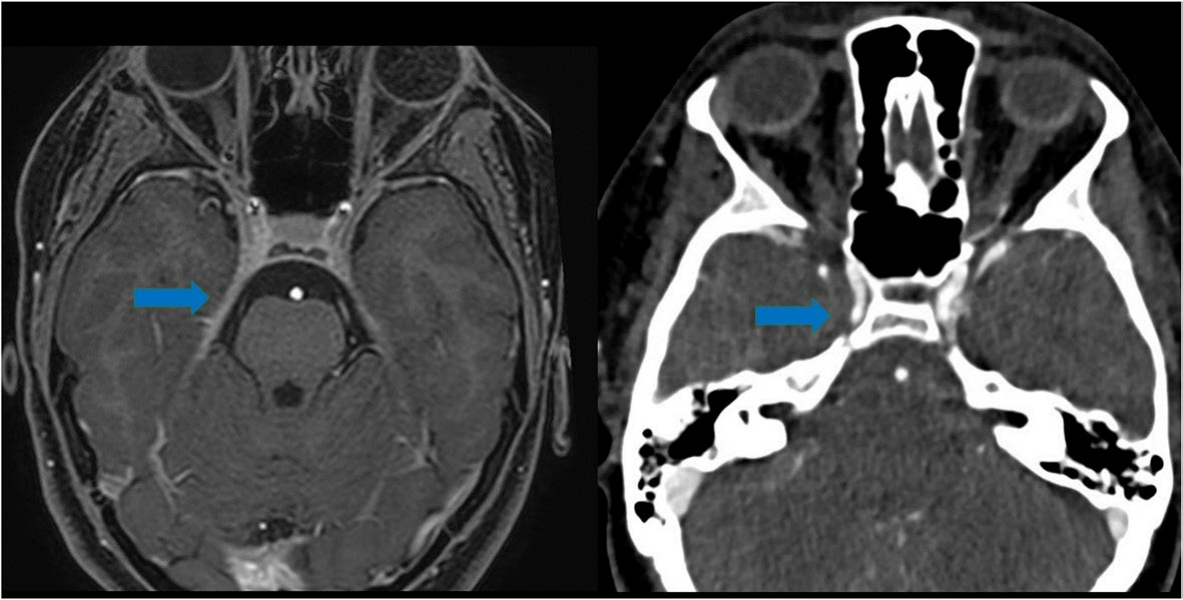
Brain MRI (left, axial T1-weighted with gadolinium) showed linear thickening of the right tentorial profile (blue arrow) in the whole segment starting from the anterior insertion and concomitant thickening of the lateral wall of the cavernous sinus. CT angiography (right) confirmed abnormal blood vessel thickening in the same region (blue arrow)
